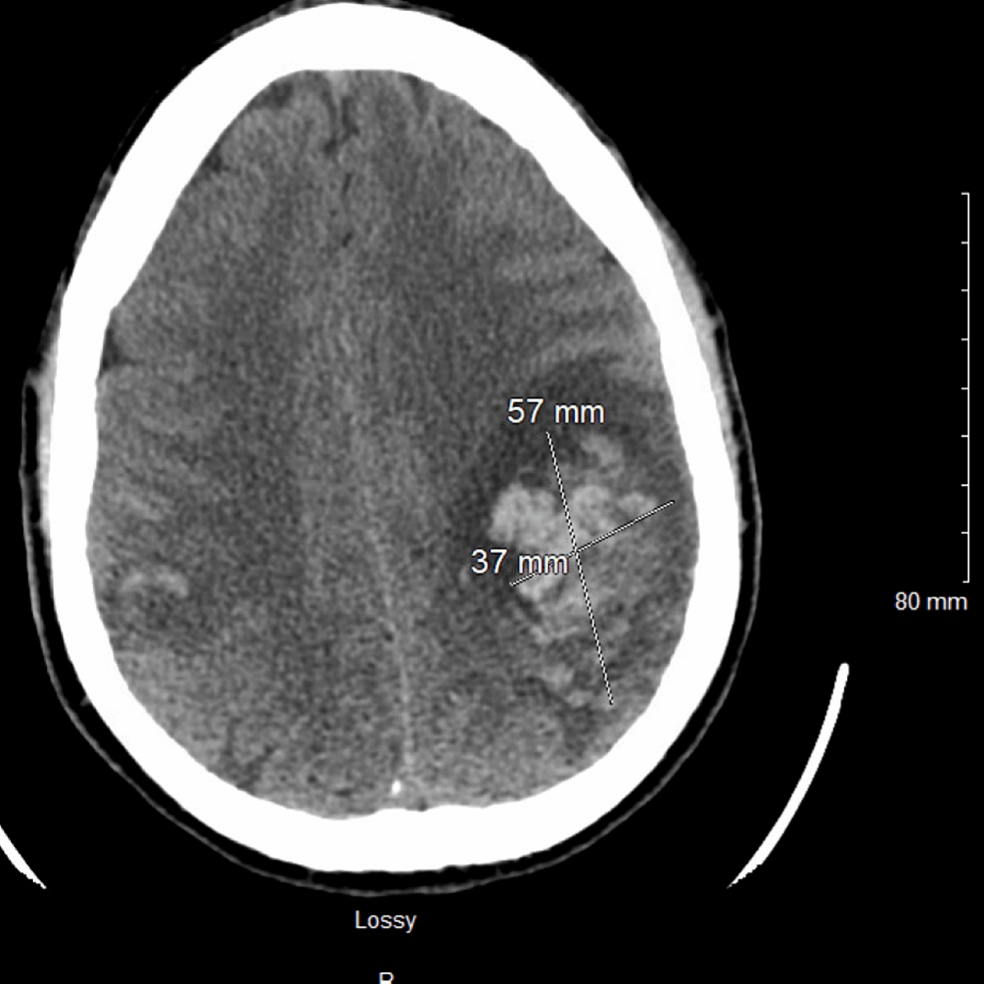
Computed tomography of the head (axial view). Left frontoparietal intraparenchymal hematoma measuring 37 mm × 57 mm.
